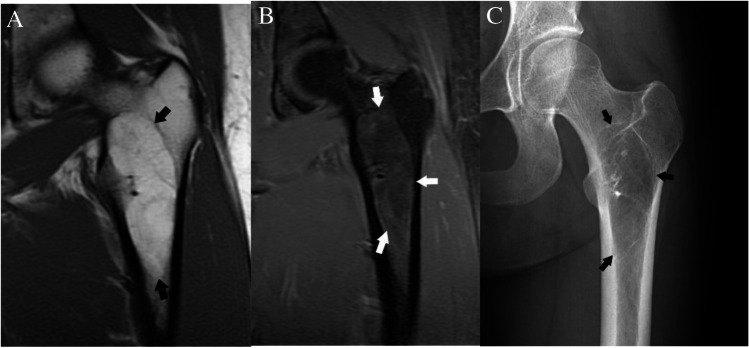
Case 1. Intraosseous lipoma. 23-year-old female runner presents with 3 months of left hip pain radiating to the groin with incidental lesion (arrows) detected in the proximal femur on MRI. The lesion is hyperintense (similar to subcutaneous fat) on the A coronal T1-weighted MR image and is hypointense on the B coronal T2-weighted fat-suppressed MR image. C Radiograph shows a lucent lesion in the proximal femur
